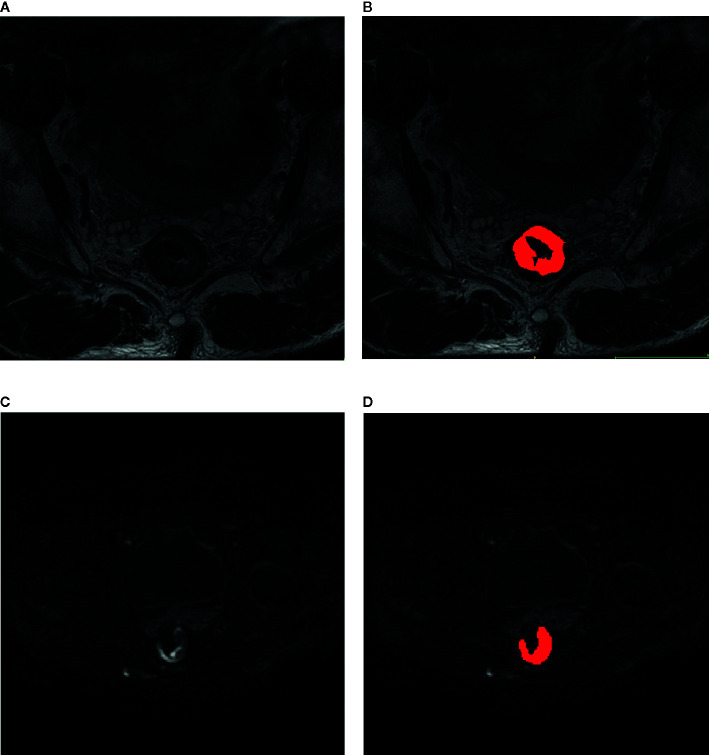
Delineation of region of interest on the images of rectal cancer. (A) T2-weighted image; (B) T2-weighted image overlaid by the manual delineation. (C) diffusion-weighted image with b-value of 1000 sec/mm^2^. (D) Diffusion-weighted image with b-value of 1000 sec/mm^2^ overlaid by the manual delineation.
